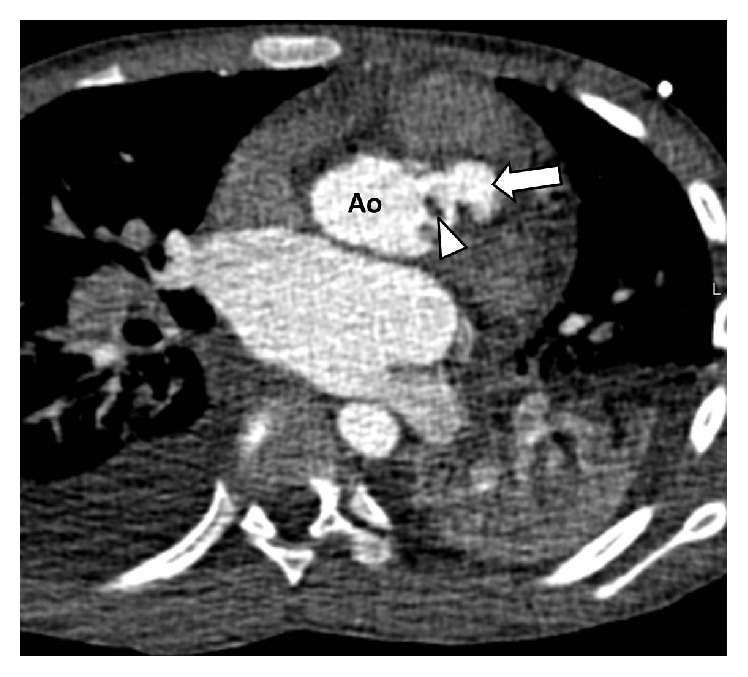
Coronal maximum intensity projection computed tomography of the aorta (Ao) showing a paravalvular mycotic pseudoaneurysm stemming from the aortic root (arrow).
